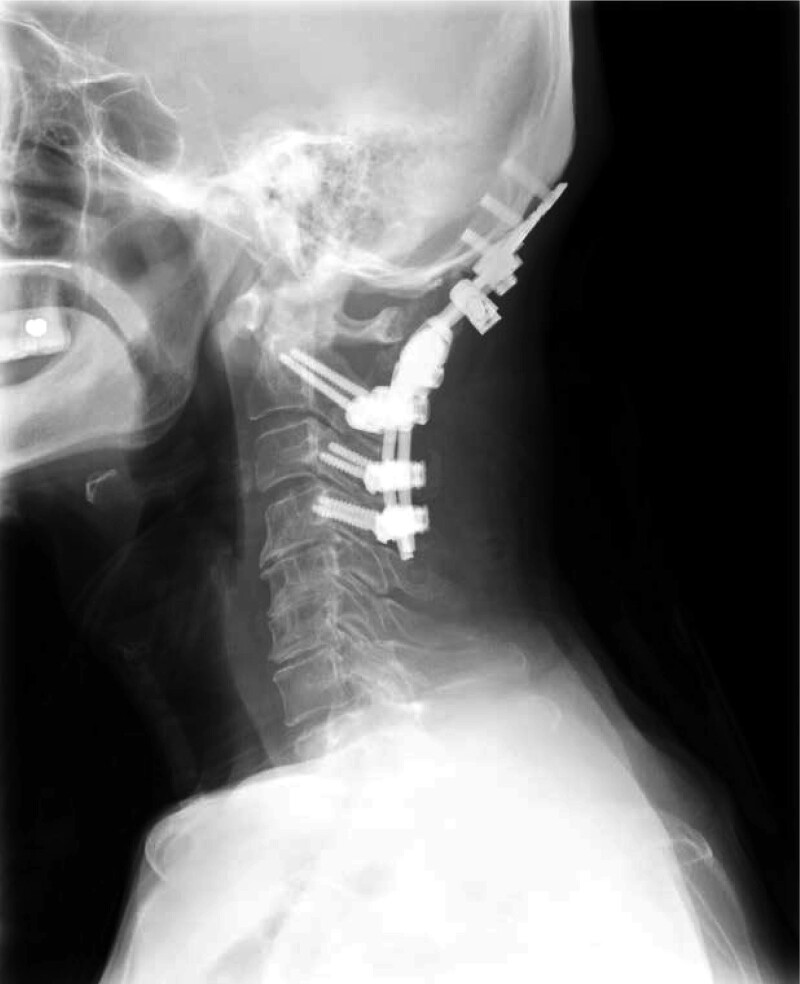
Postoperative radiology. Radiograph at 2 weeks postoperatively shows that the implants are stable without loosening.
